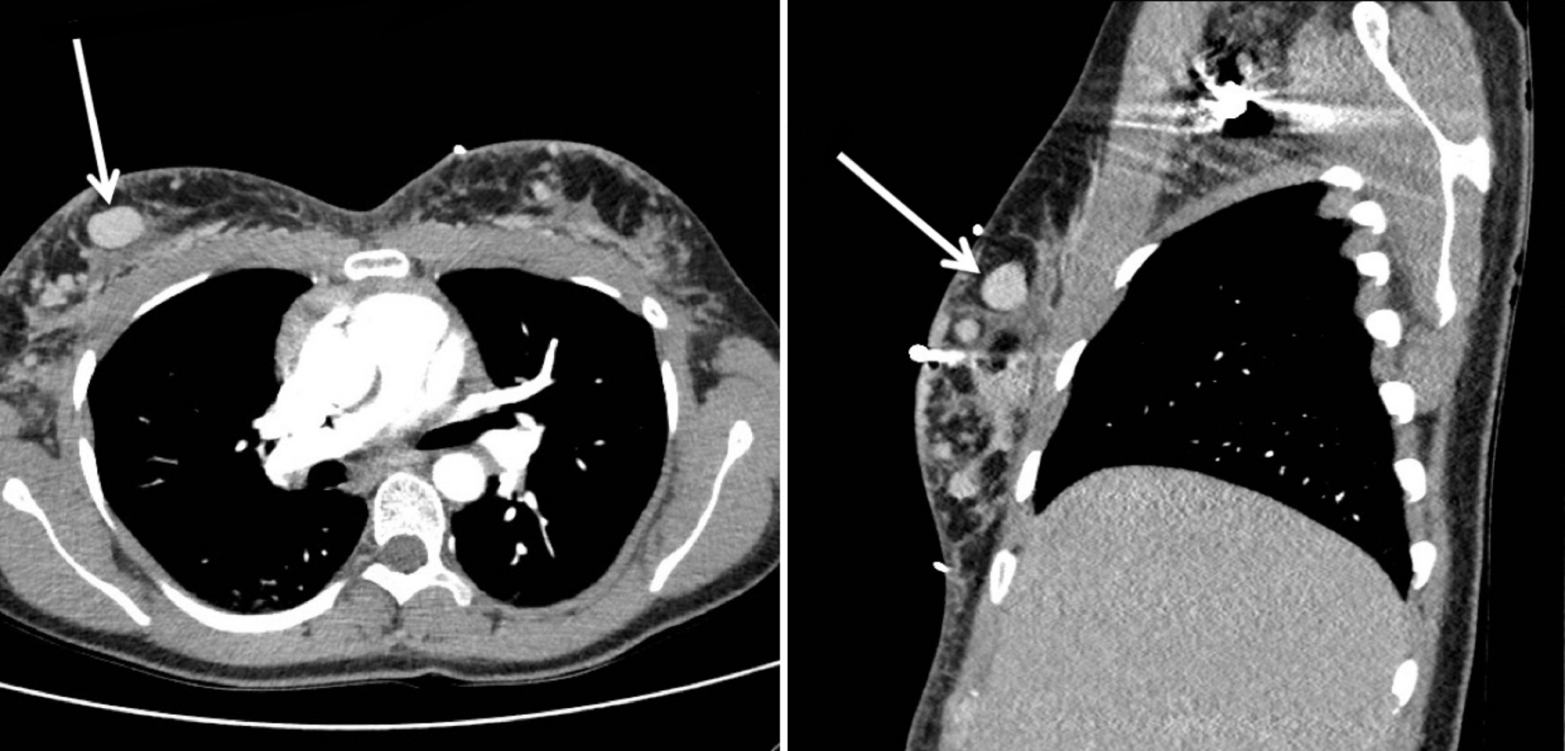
CT Chest Axial* (left)* and sagittal *(right) *contrast-enhanced CT images of the chest demonstrate bilateral areas of prior free-silicone injection with surrounding granulomatous change. The largest globule of free silicone is visualized within the right breast *(arrows)*.
